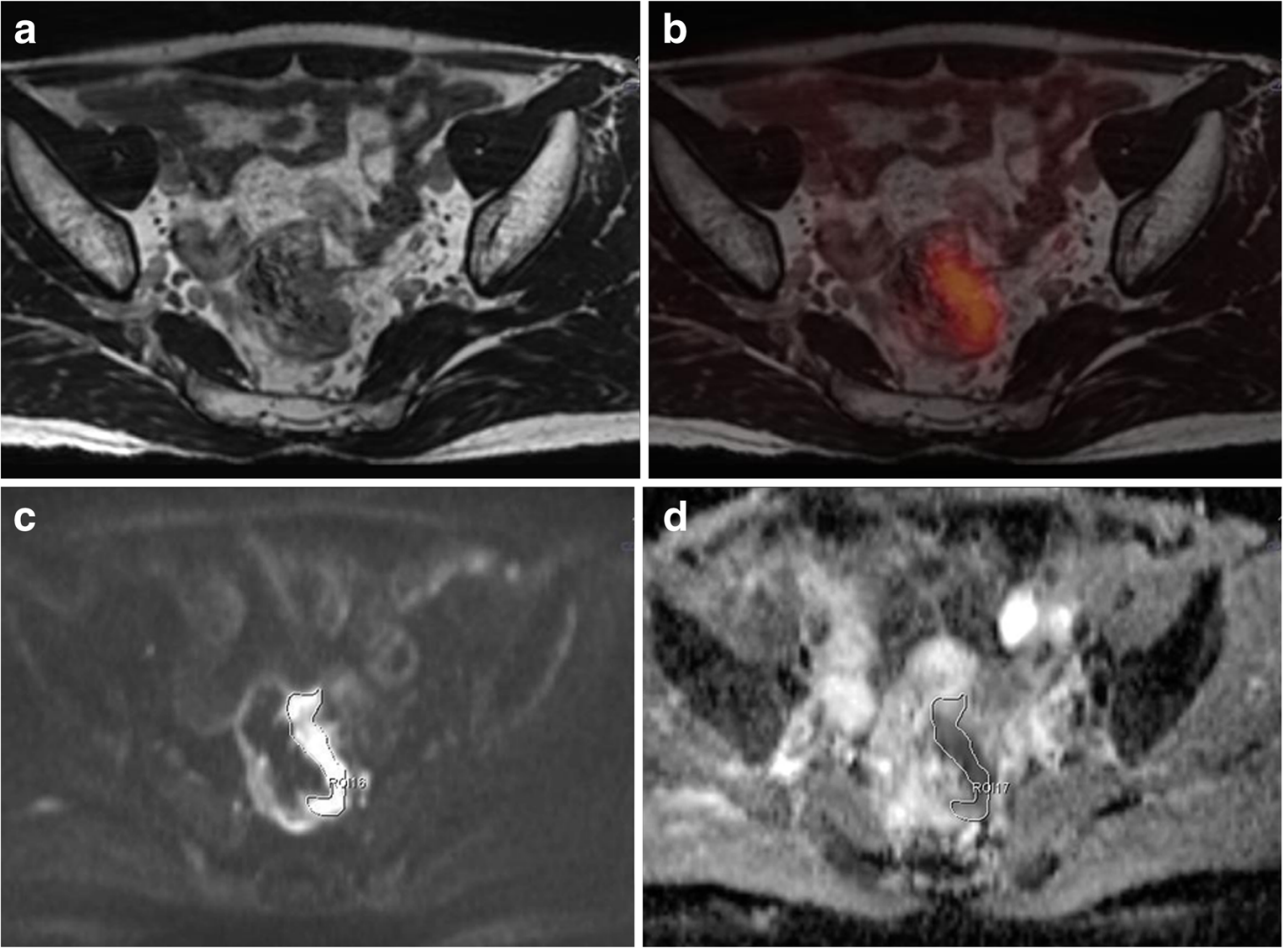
A 58-year-old man with rectal adenocarcinoma. Axial T2-weighted SPACE image (a), fused ^18^ F-FDG PET image and axial T2-weighted SPACE image (b) obtained by hybrid PET/MR showing FDG avid rectal wall thickening at the left lateral wall. SUVmax, SUVpeak, and SUVmean of the tumor were 8.14, 6.76, and 5.21, respectively. DWI (c) depicted the tumor as a high signal intensity lesion, and the corresponding ADC map (d) showed reduced ADC in the tumor (ADCmean was 1.04 × 10^−3^ mm^2^/s)
